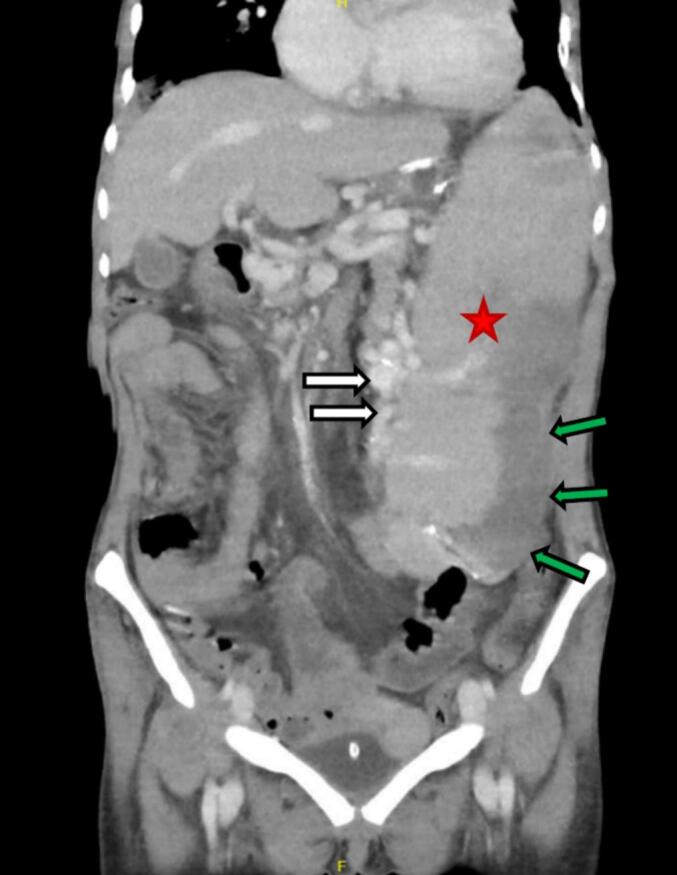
Pre-operative CT of the abdomen and pelvis demonstrating massive splenomegaly (red star) with associated splenic hilar and lienorenal varices (white arrows) and infarcted inferolateral area of the spleen (green arrows). Patient has a history of malaria and left-sided portal hypertension with varices.
